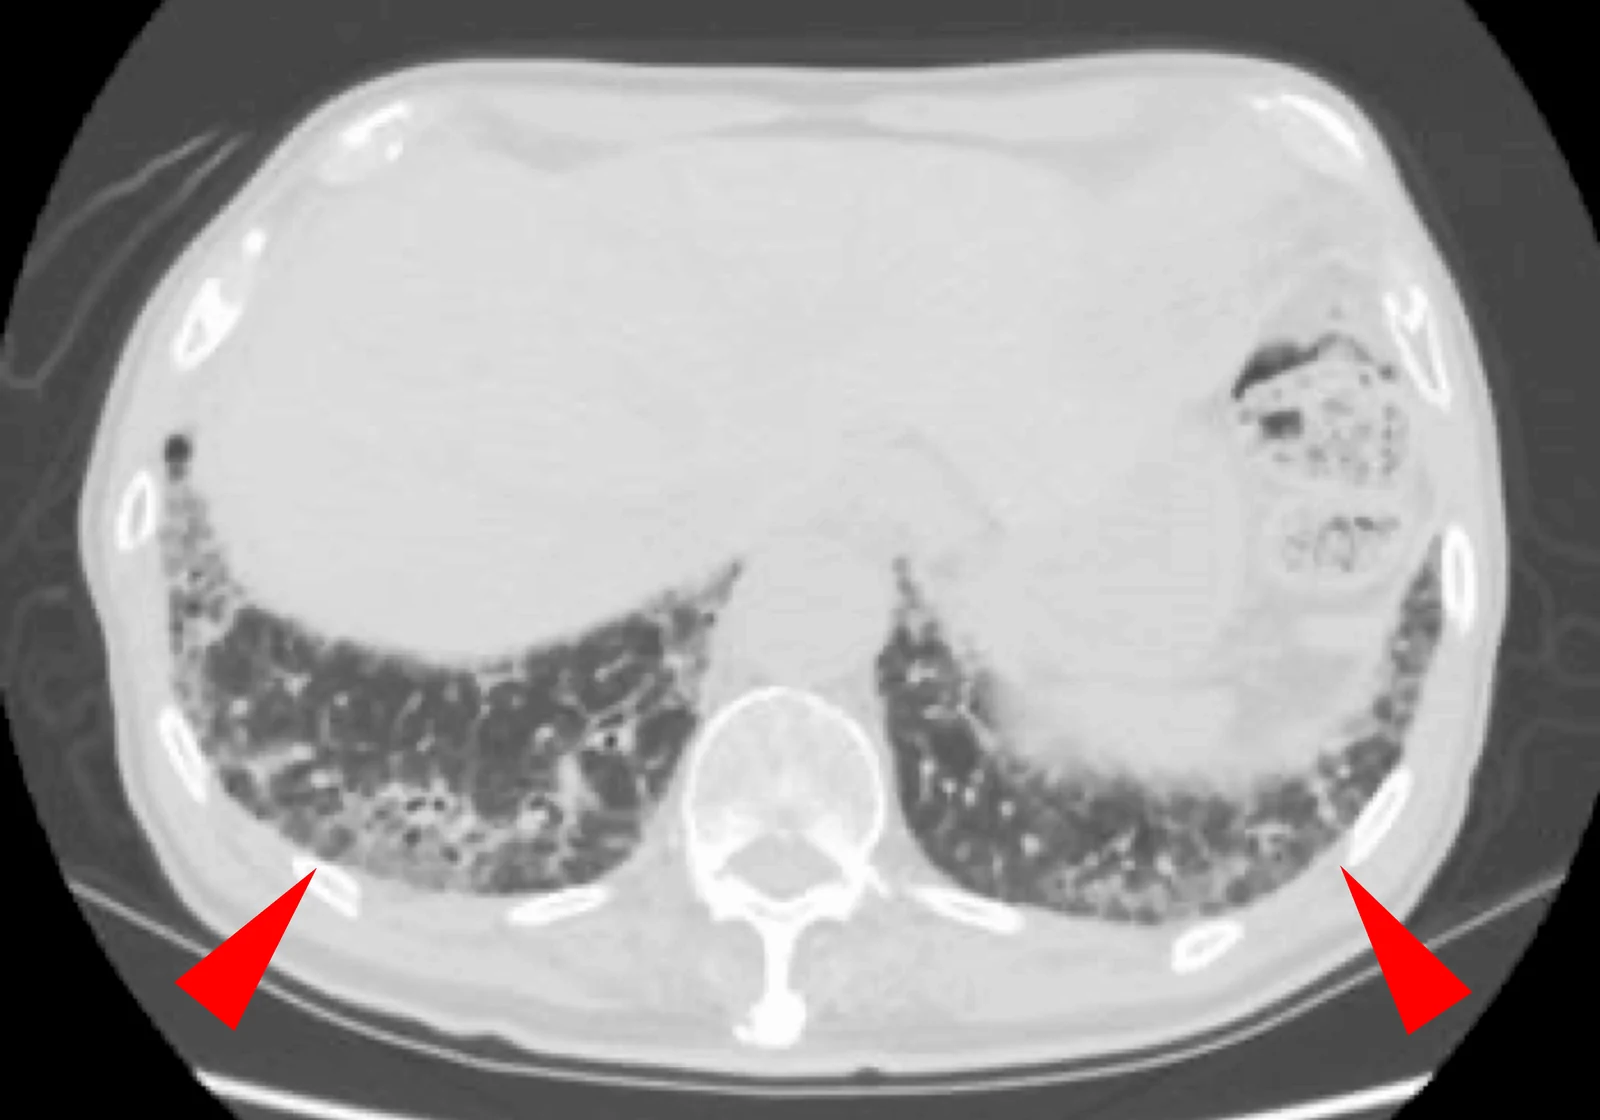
Chest computed tomography performed three years before admission Chest computed tomography demonstrating bilateral subpleural reticular opacities and fibrotic changes, highlighted by red arrowheads
